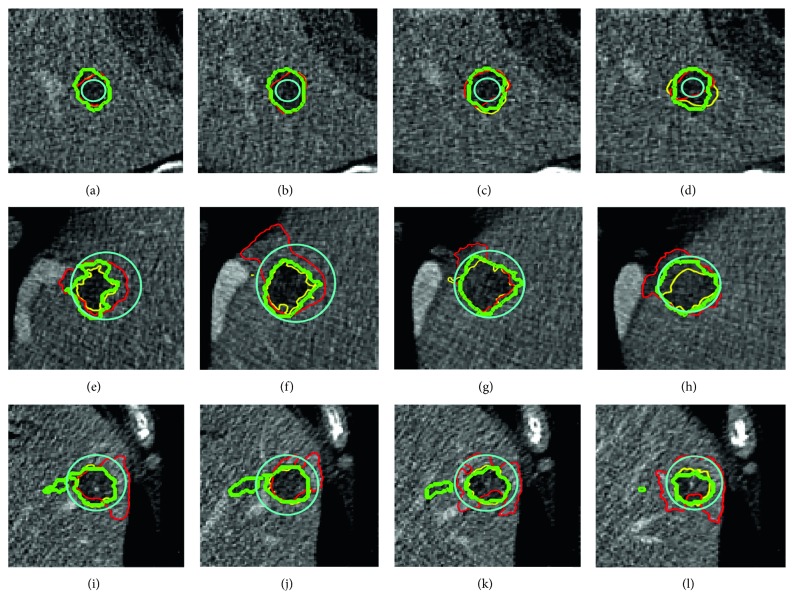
3D liver tumor segmentation using DRLS and FLS in comparison. (a)–(d), (e)–(h), and (i)–(l) are adjacent slices of three 3D CT scans. (a)–(d) are located inside the tumor, and the other two are close to or outside the tumor boundary. Cyan: initial contour; green: manual radiologists' annotation; red: FLS result; yellow: our proposed method.
